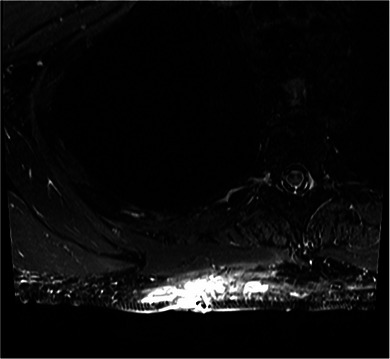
Exemplary image from the MRI‐scan performed nine days after inpatient admission
